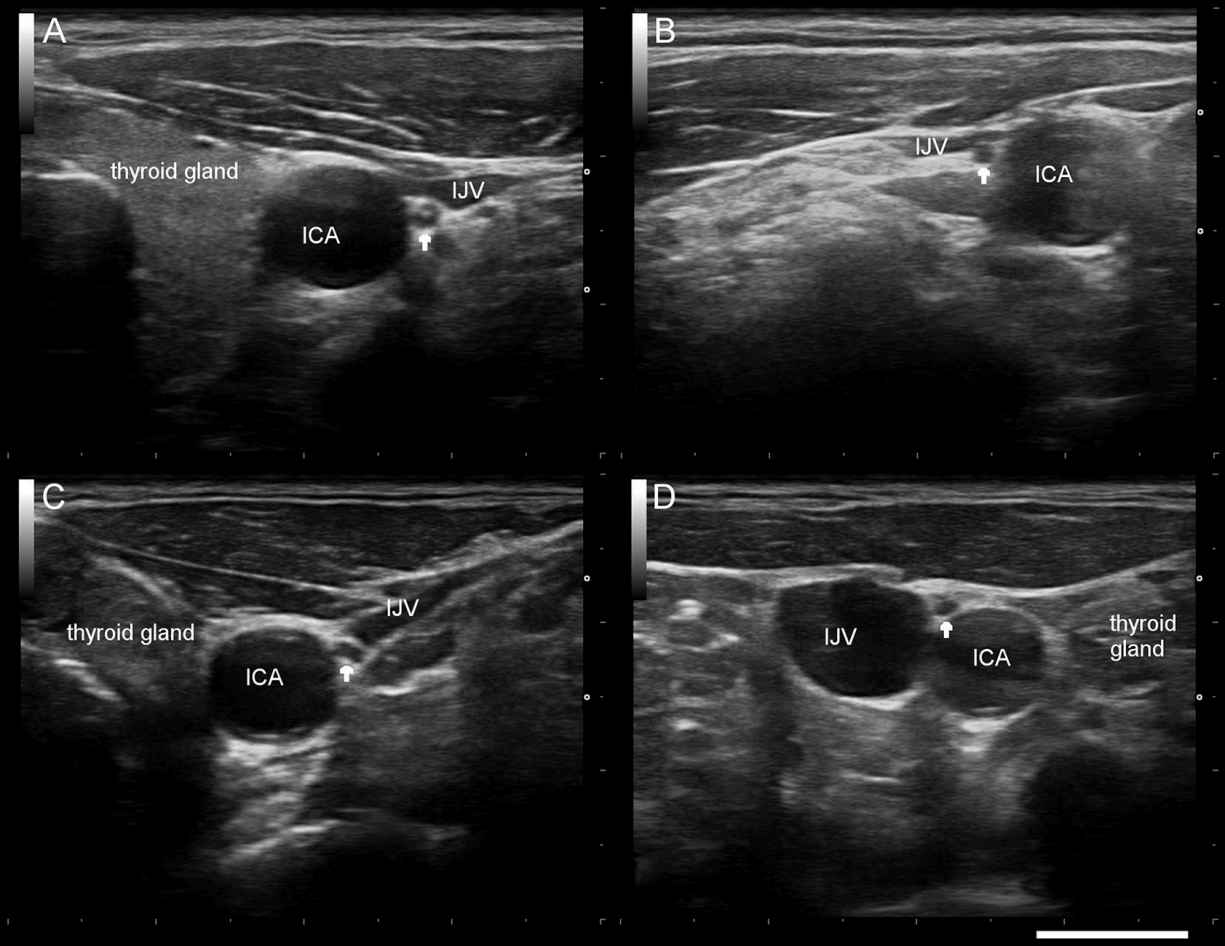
Typical sonographic findings of the right/left vagus nerve (arrow) in a healthy control subject (A,B) and a patient with Parkinson's disease (C,D). *ICA* internal carotid artery, *IJV* internal jugular vein. Scale bar 1 cm.
